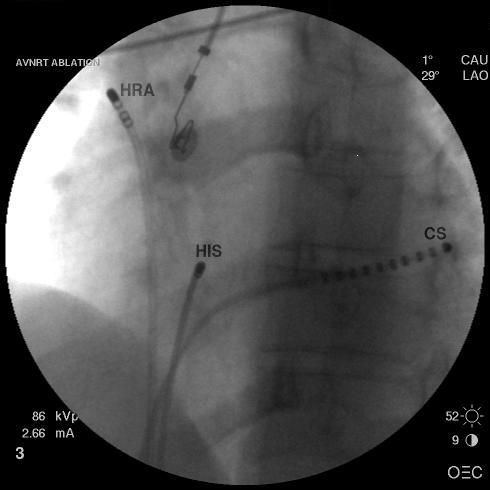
Left anterior oblique radiograph of catheters position during measurements
